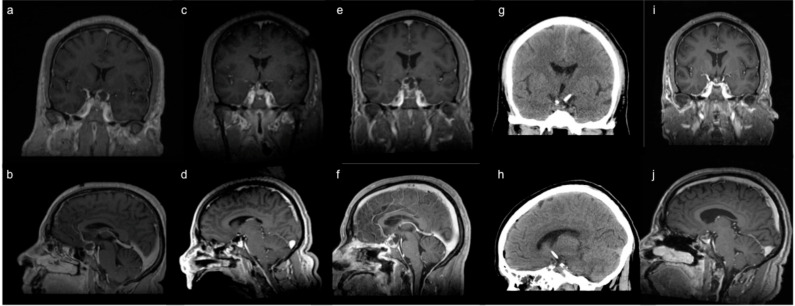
Ommaya reservoir placement for cystic recurrence. A 52-year-old male was diagnosed with a cystic suprasellar lesion on work-up for progressive left sided visual deficits, consistent with craniopharyngioma. Representative (a) coronal and (b) sagittal T1-weighted post-contrast MRI showed a predominantly cystic, enhancing 1.5 cm suprasellar lesion compressing the optic nerves, eccentric to the left. Intra-operative (c) coronal and (d) sagittal T1-weighted post-contrast MRI after resection demonstrated decompression of the optic chiasm and nerves with known adherent residual tumor left intentionally along the cavernous sinus. (e) Coronal and (f) sagittal T1-weighted post-contrast MRI obtained 5 months after initial resection showed cystic recurrence in the setting of recurrent visual decline. This was treated with Ommaya reservoir placement, as depicted on (g) coronal and (h) sagittal post-operative head CT, followed by adjuvant stereotactic radiosurgery. (i) Coronal and (j) sagittal T1-weighted post-contrast MRI obtained 3 months after cyst drainage and adjuvant radiation demonstrated durable cyst decompression with improvement of the patient’s visual symptoms.
